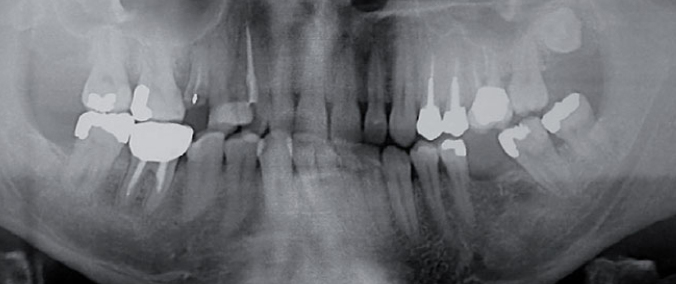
A panoramic radiograph of a 34-year-old male patient presenting widespread caries and several elements which need to be removed.
